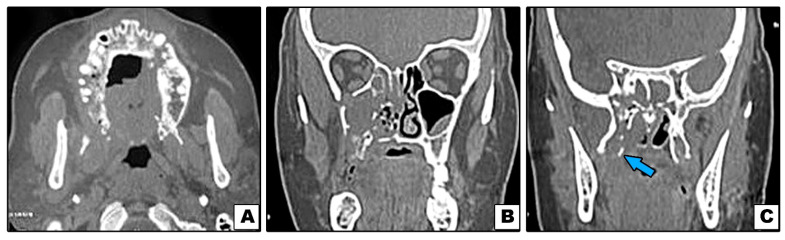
Case#13: (A–C) Axial and coronal CT showing bone destruction involving premaxilla, right maxilla with pterygoid plates (arrow), and not involving inferior orbital rim.
